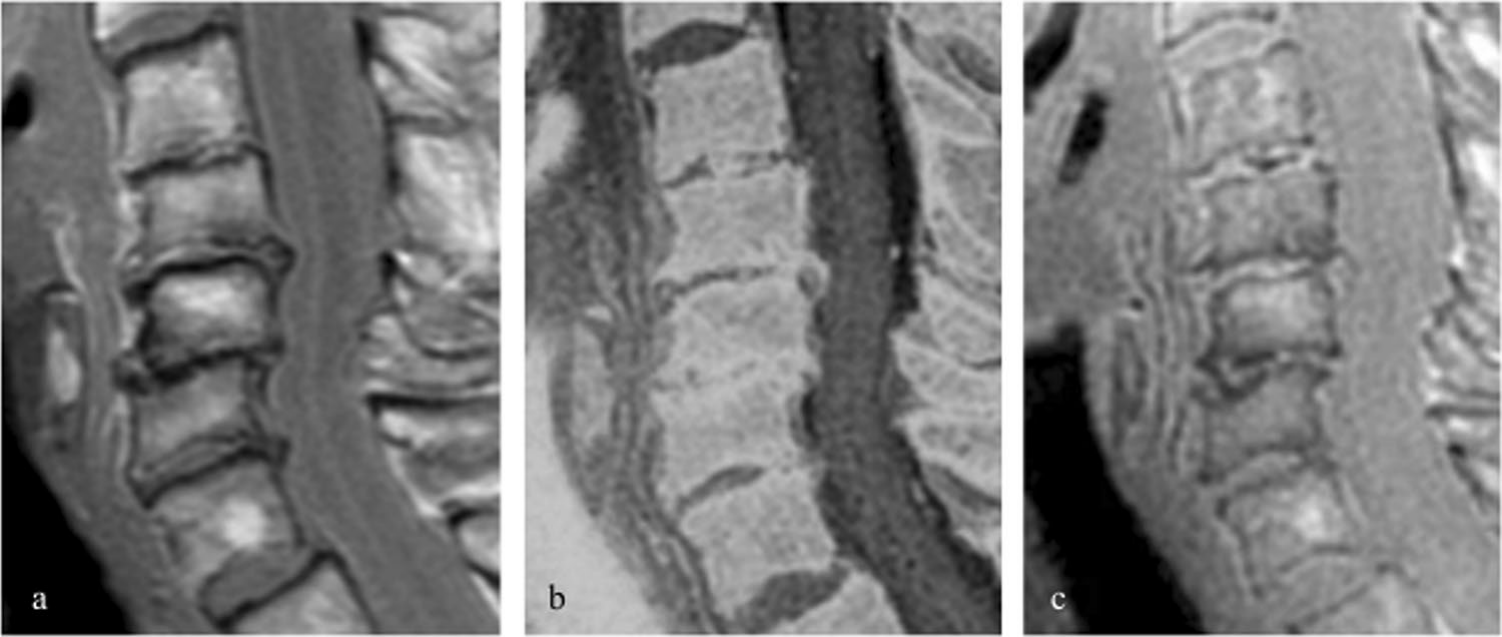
Imaging of the cervical spine: Exemplary images in sagittal plane on standard T1-weighted TSE (a), FRACTURE (b) and UTE (c) sequence of a degenerative altered cervical spine. Note significant productive changes with posterior spondylophytes, endplate sclerosis and slight retrolisthesis in mid-cervical segments
